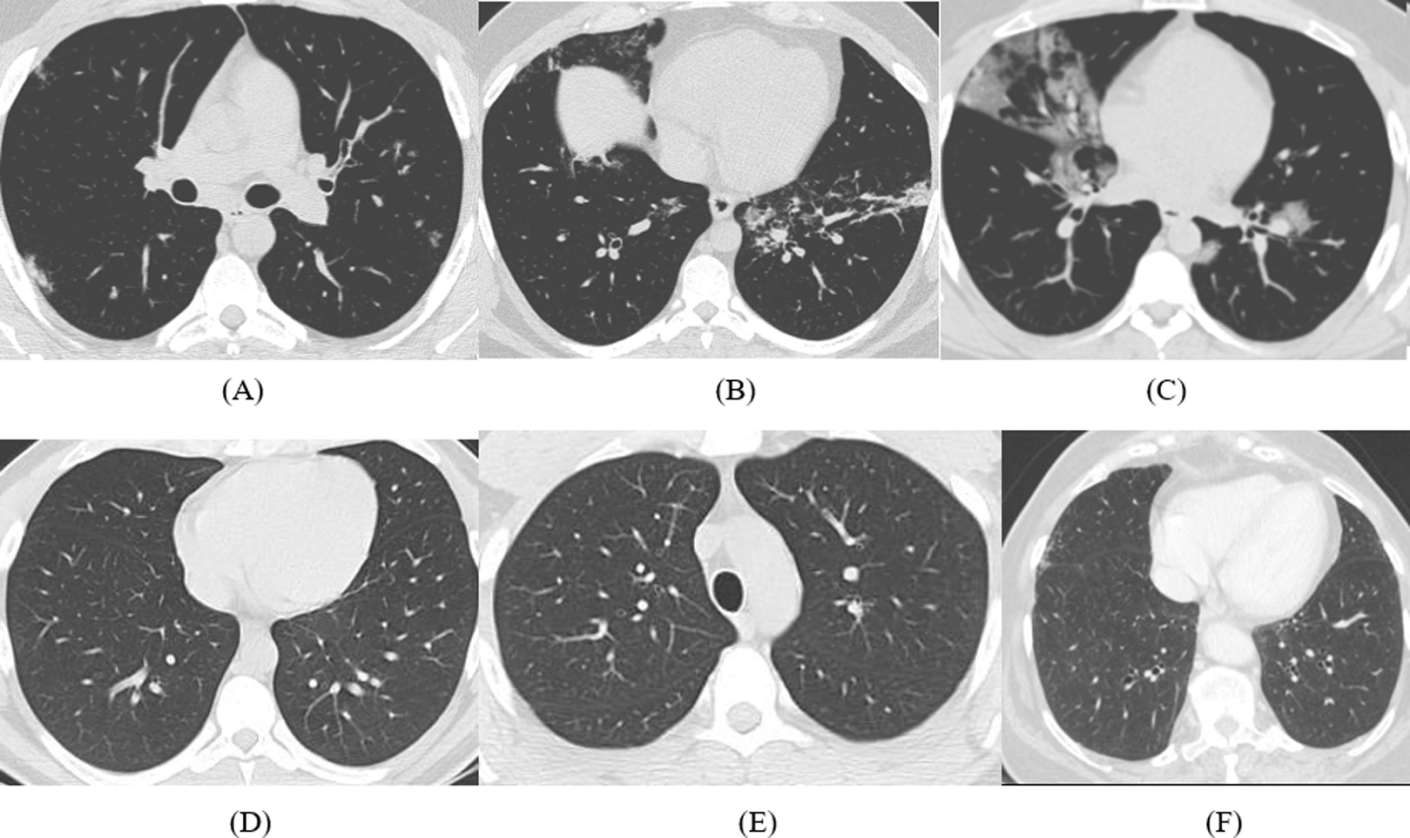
Samples of CT images (A)–(C) COVID-19 CT images and (D)–(F) non-COVID-19 CT images.
